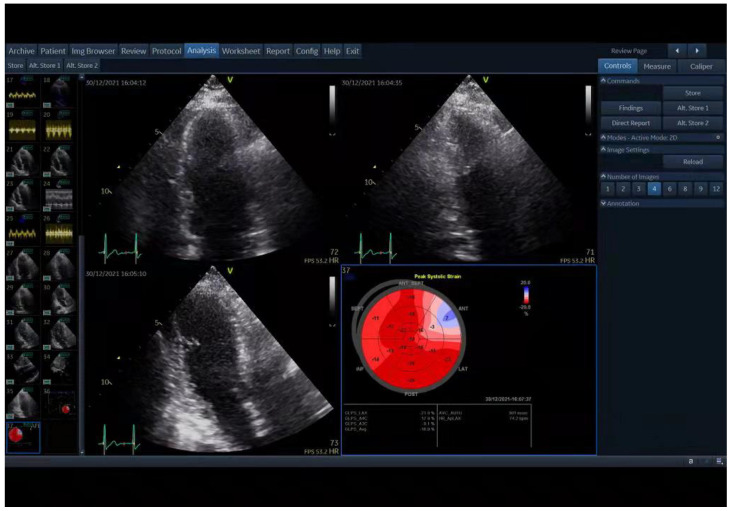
Two-dimensional speckle-tracking echocardiography (2D STE) analysis shows the result of global longitudinal strain (GLS) on a bull’s-eye depiction acquired by EchoPAC.
